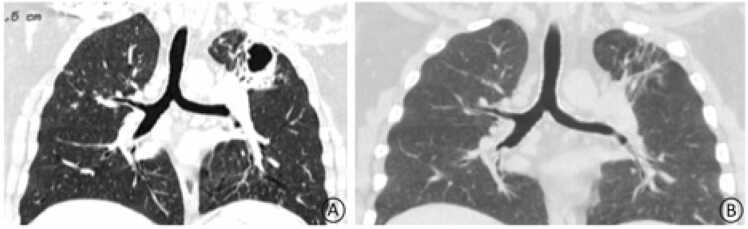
(A) CT chest demonstrating a 4.2 × 3.8 cm cavitary lesion in the left upper lobe. (B) Follow-up CT chest demonstrating marked interval improvement with only a small residual cavitary lesion remaining in the left upper lobe.
